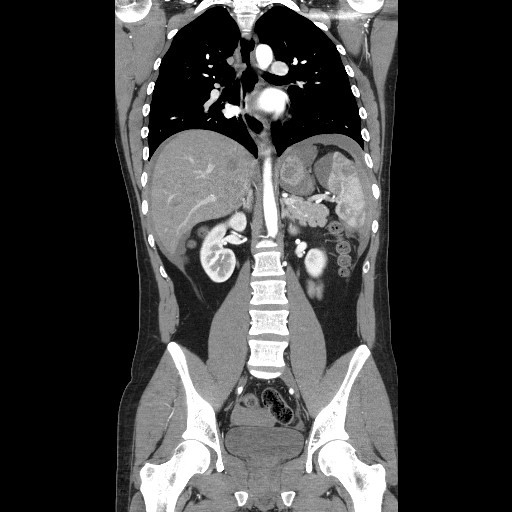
Coronal computed tomography image demonstrating macerated spleen, perisplenic hematoma and hemoperitoneum.
